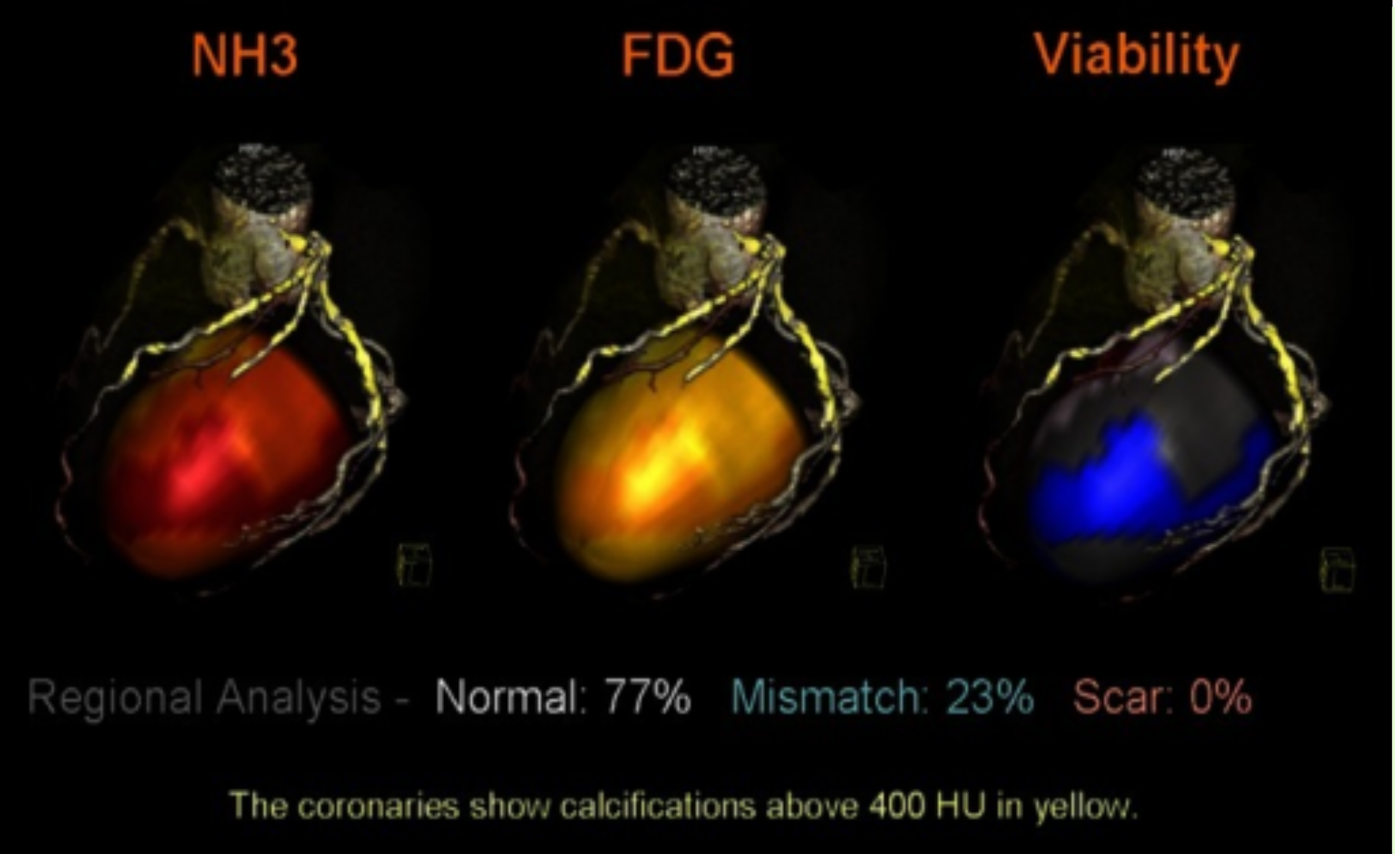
PET perfusion data mapped onto the epicardial surface of CT angiography to evaluate the viability of cardiac muscle and segmented coronary tree. The image depicts the simultaneous mapping of metabolism, coronary anatomy, and myocardial perfusion via fused images. NH3, ammonia, FDG, F-fluorodeoxyglucose, HU, Hounsefield units (23).
